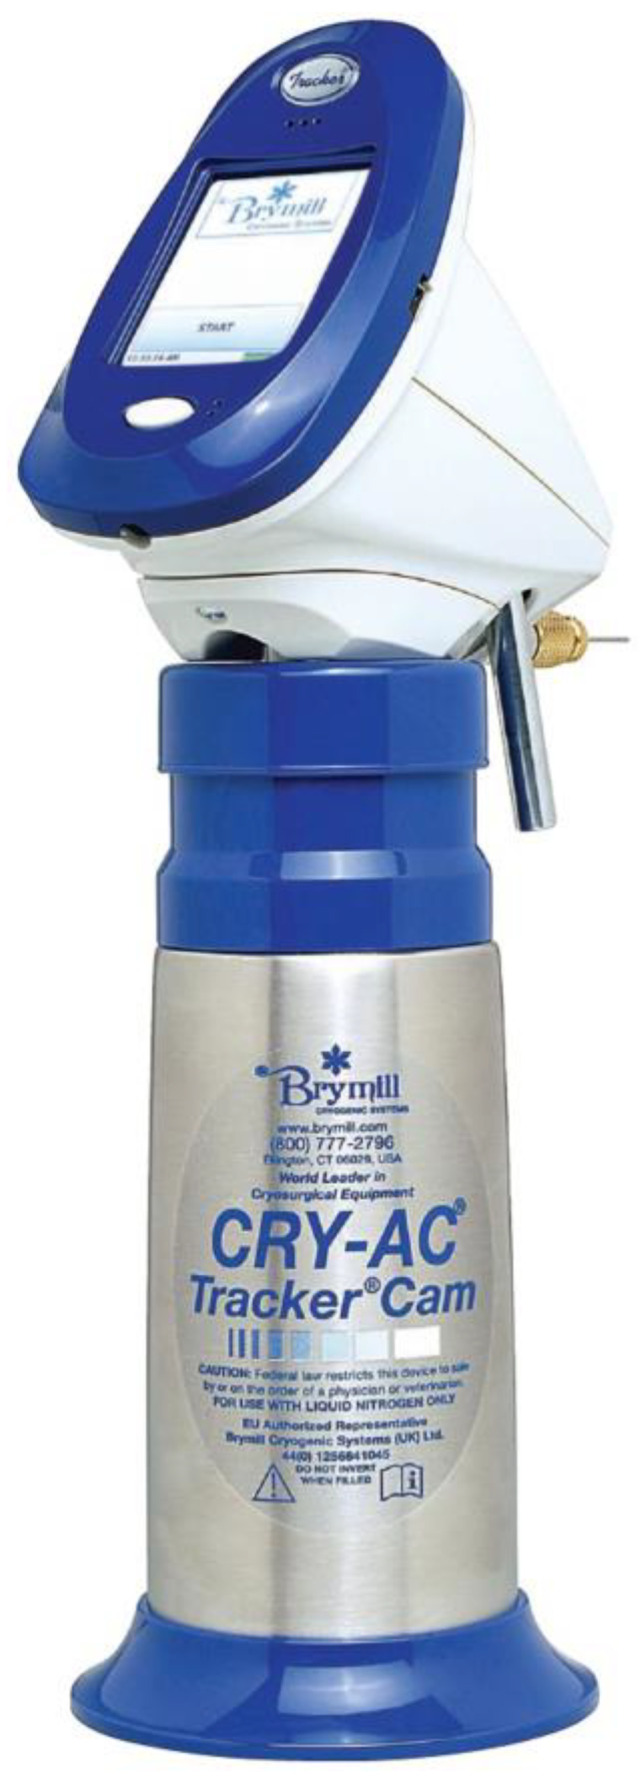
An updated cryotherapy device (Cry-Ac Tracker Cam) that measures the skin temperature when spraying liquid nitrogen on the selected skin lesion using an infrared sensor. The operator can set the device to indicate when a predetermined freeze temperature has been achieved at the lesion (courtesy of Brymill Cryogenic Systems, Ellington, CT).
